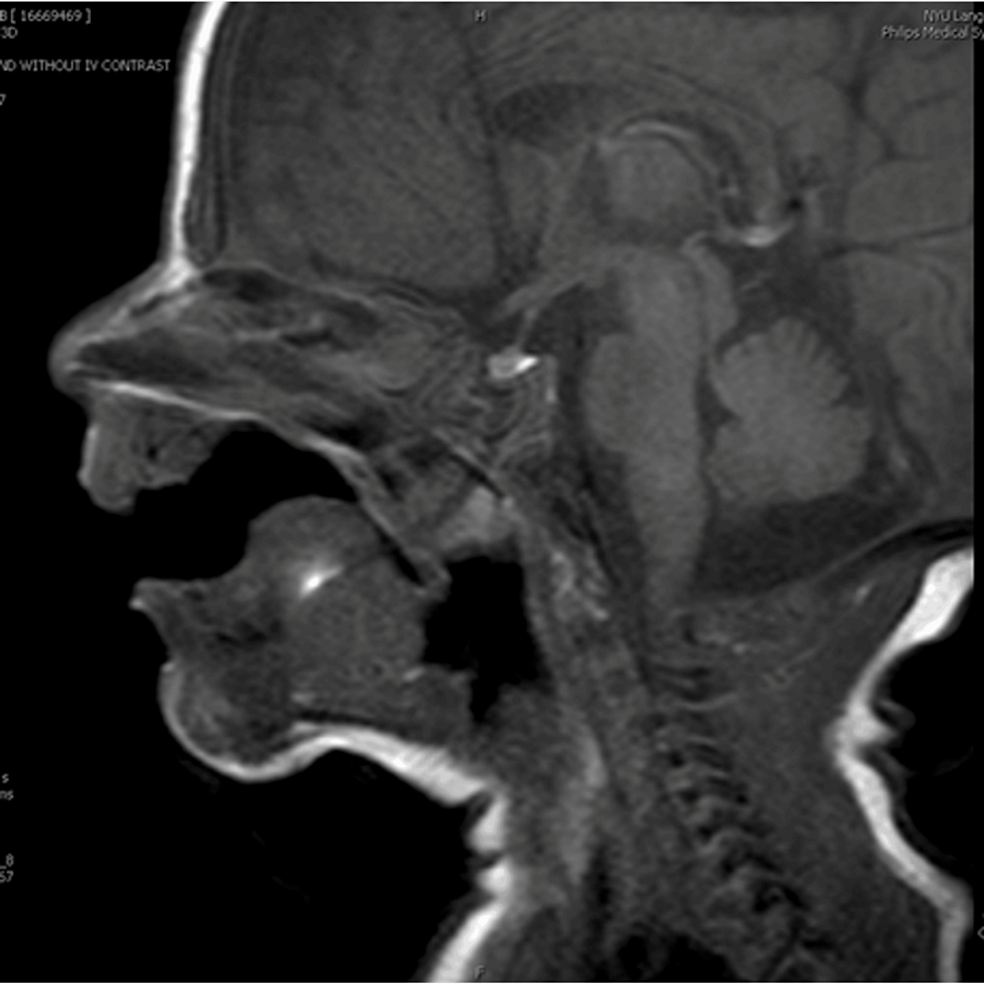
MRI face with contrast done on day of life 13 revealed similar findings of the mass with no evidence of vascularization.
